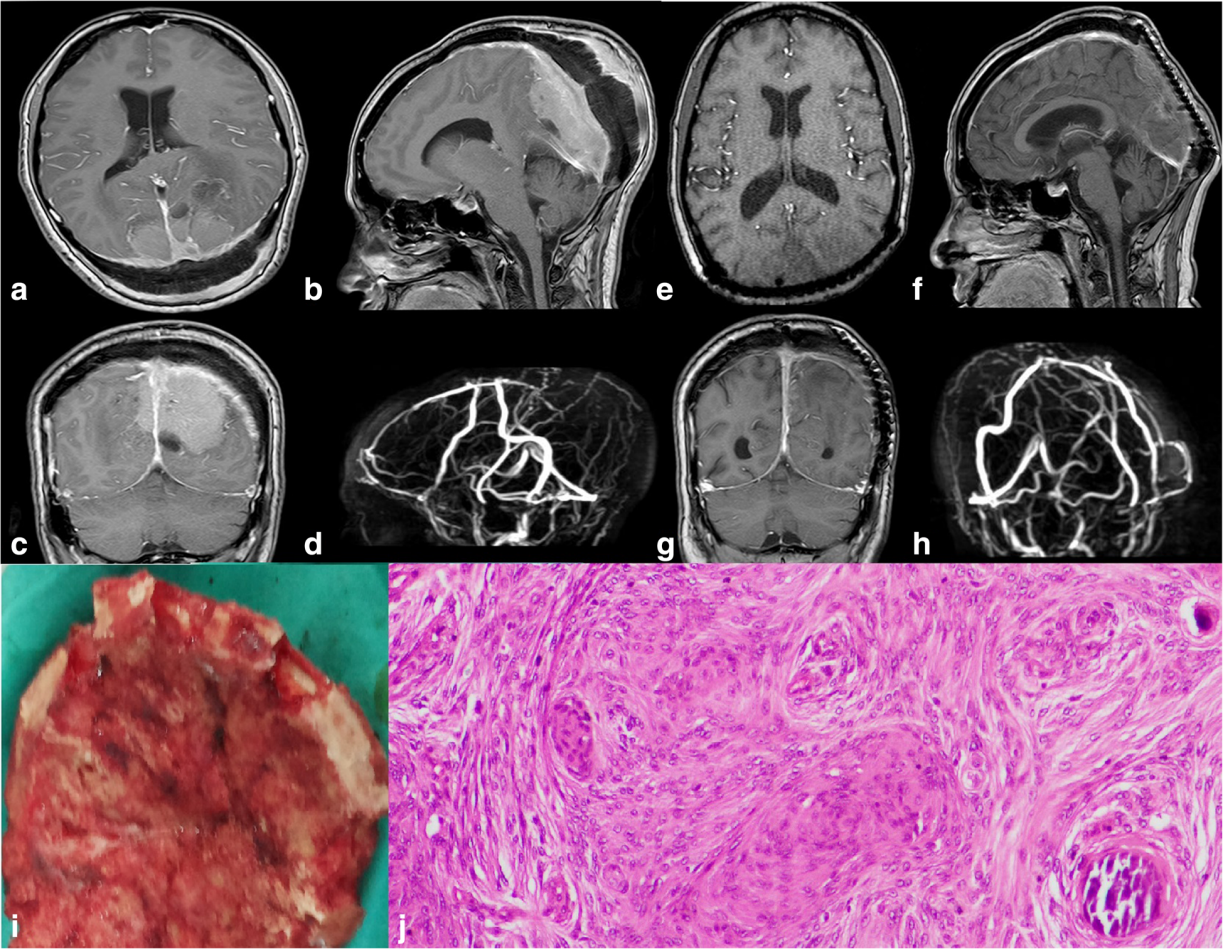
Meningioma in plaque situated in the parasagittal region. Preoperative image, MRI with contrast. a Axial view verified an enhanced occipital mass. b Sagittal view showed that tumor attached to the bone and involved the sagittal sinus. c Coronal view demonstrated mass lesion was crossing the midline bilateral in the parieto-occipital region more on the left side. The tumor was surrounded by mild edema. d It implicated the posterior aspect of the superior sagittal sinus and left transverse sinus in venography. Two year’s postoperative MRI with contrast. e Axial view revealed complete removal with nearly no mass lesion and decompressed occipital horn. f Sagittal view showed small residual mass parafalcine encasing superior sagittal sinus; the bony defect was closed by titanium mesh. g In coronal view, we discovered no mass lesion in the parieto-occipital region. h The posterior aspect of the superior sagittal sinus was opened. Geriatric Scoring System (GSS) score = 18. i It showed gross bony invasion of the tumor. j Slide represented syncytial meningioma with scatted psammoma bodies, WHO grade I
